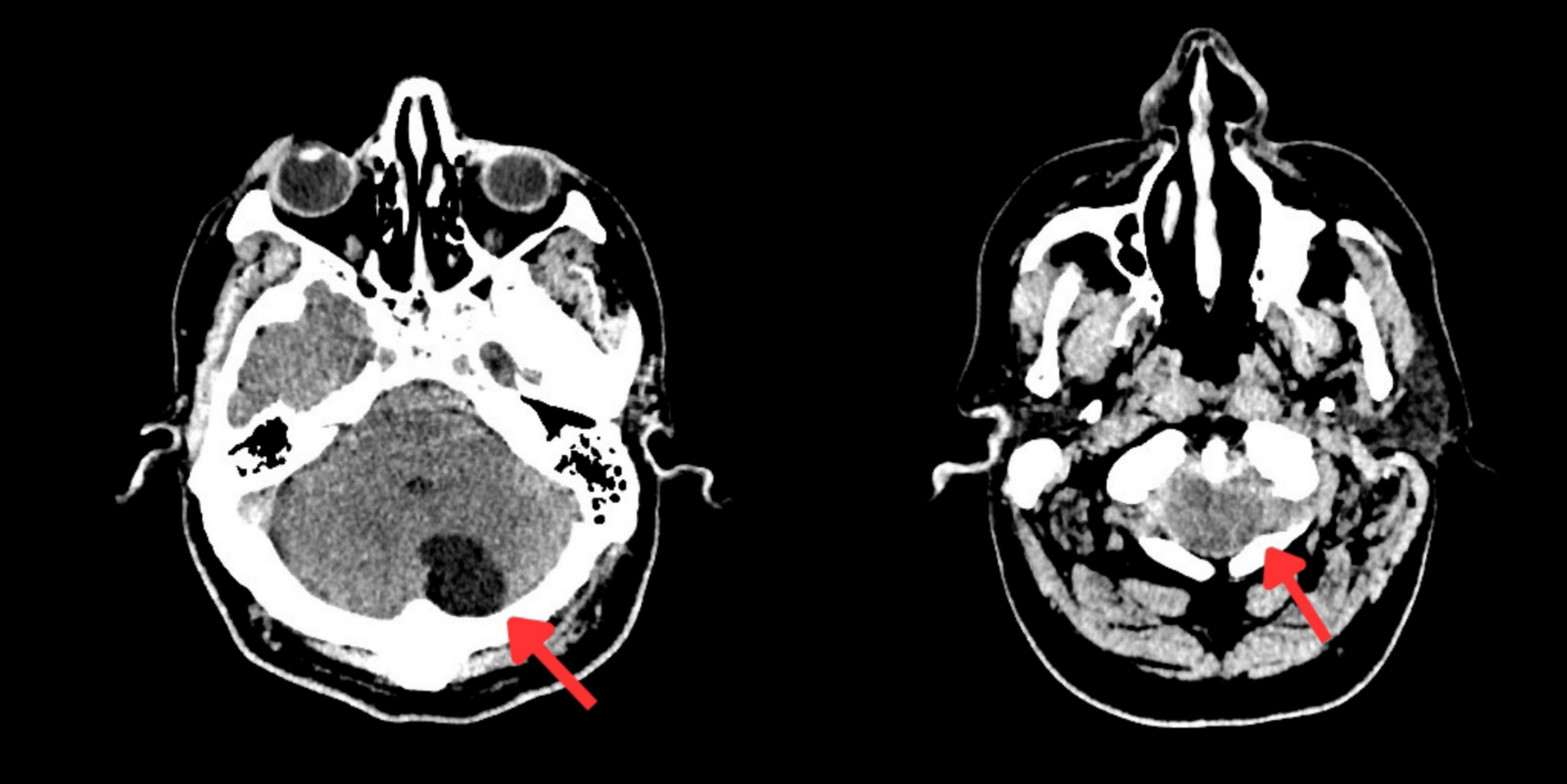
Non-contrast computed tomography (NCCT) head shows cerebellar cyst and tonsillar ectopia (indicated by arrows)
